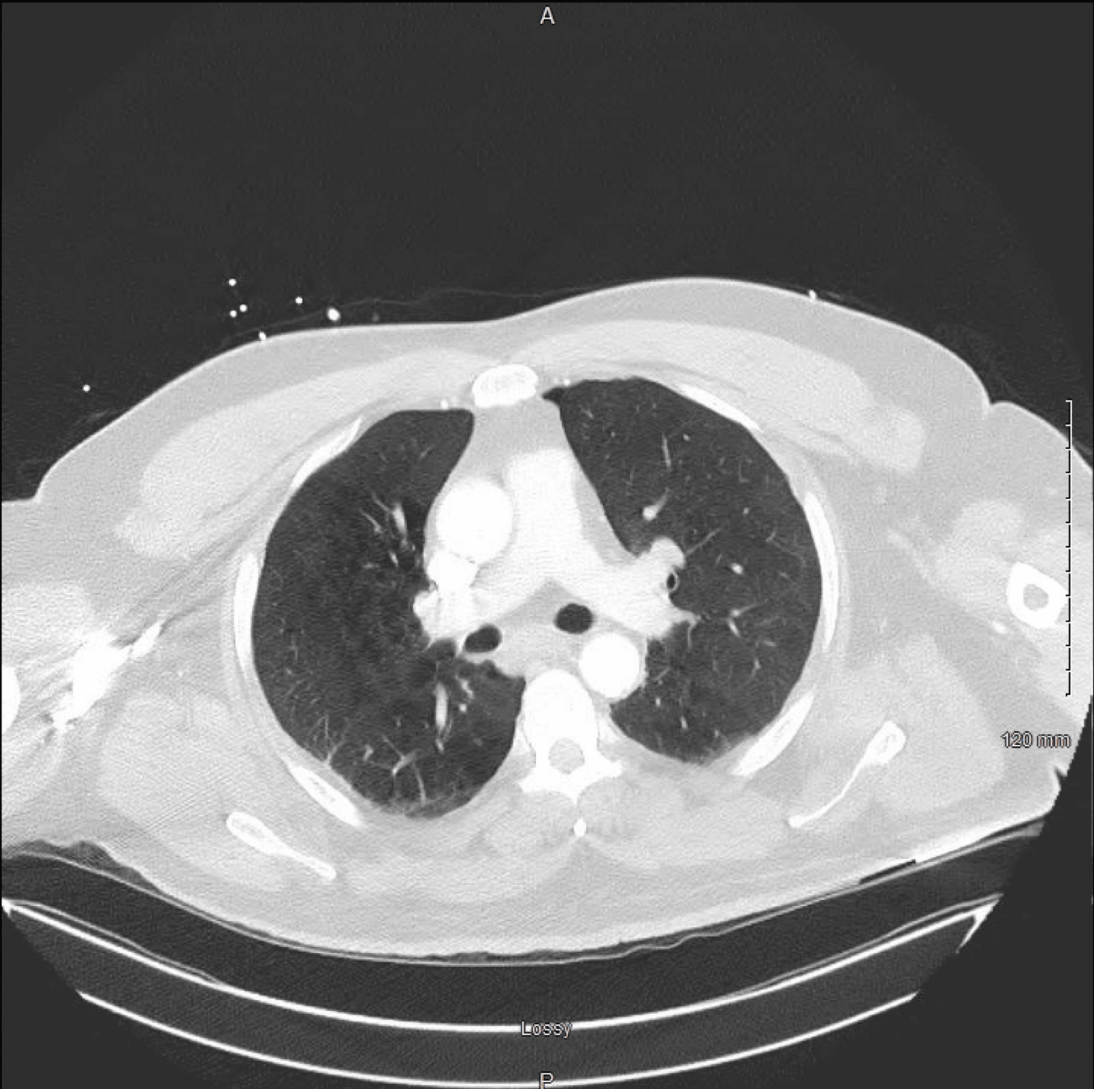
Chest CT scan A chest CT scan ordered initially to exclude the presence of pulmonary embolism. Notable in the picture are emphysematous changes
